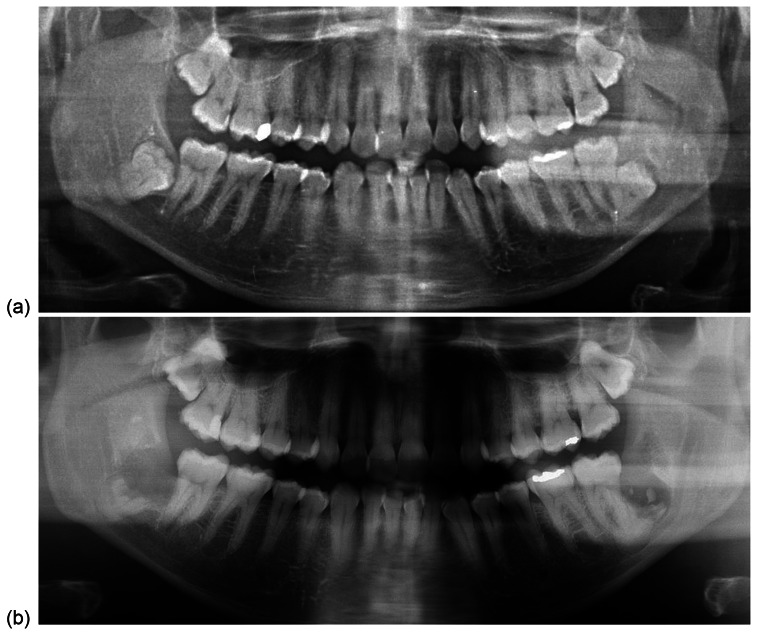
Pre-operative (a) and post-operative (b) orthopantomograms of Case 1
